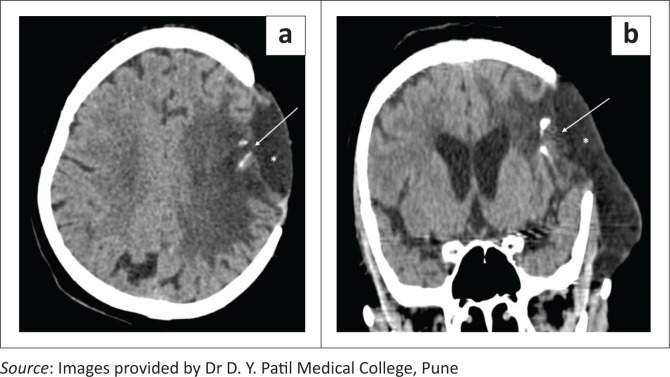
External brain tamponade. Axial (a) and reformatted coronal soft tissue window (b) CT images in a patient with a left fronto-parietal craniotomy demonstrate a large hypodense subgaleal fluid collection causing compression of the underlying left frontoparietal brain parenchyma (long arrows).
